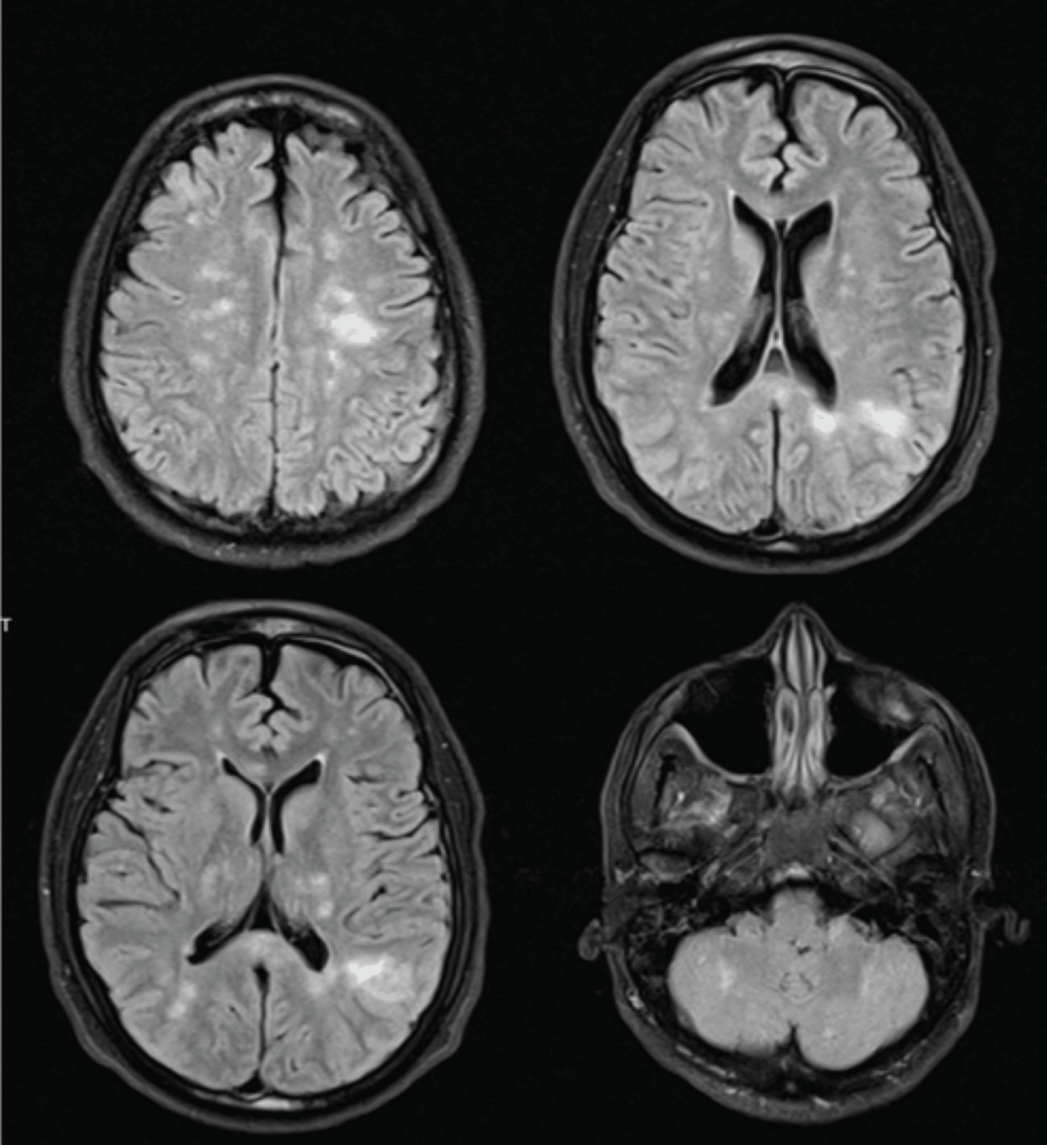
T2-FLAIR magnetic resonance imaging (MRI) showing numerous foci of hypointensity in the cerebral and cerebellar hemispheres consistent with microbleeds. Also showing multiple foci of T2/FLAIR hyperintensity within subcortical white matter and bilateral thalami, corona radiata and centrum semiovale; as well as T2/FLAIR hyperintensity within the cerebellar white matter FLAIR: fluid-attenuated inversion recovery; MRI: magnetic resonance imaging
